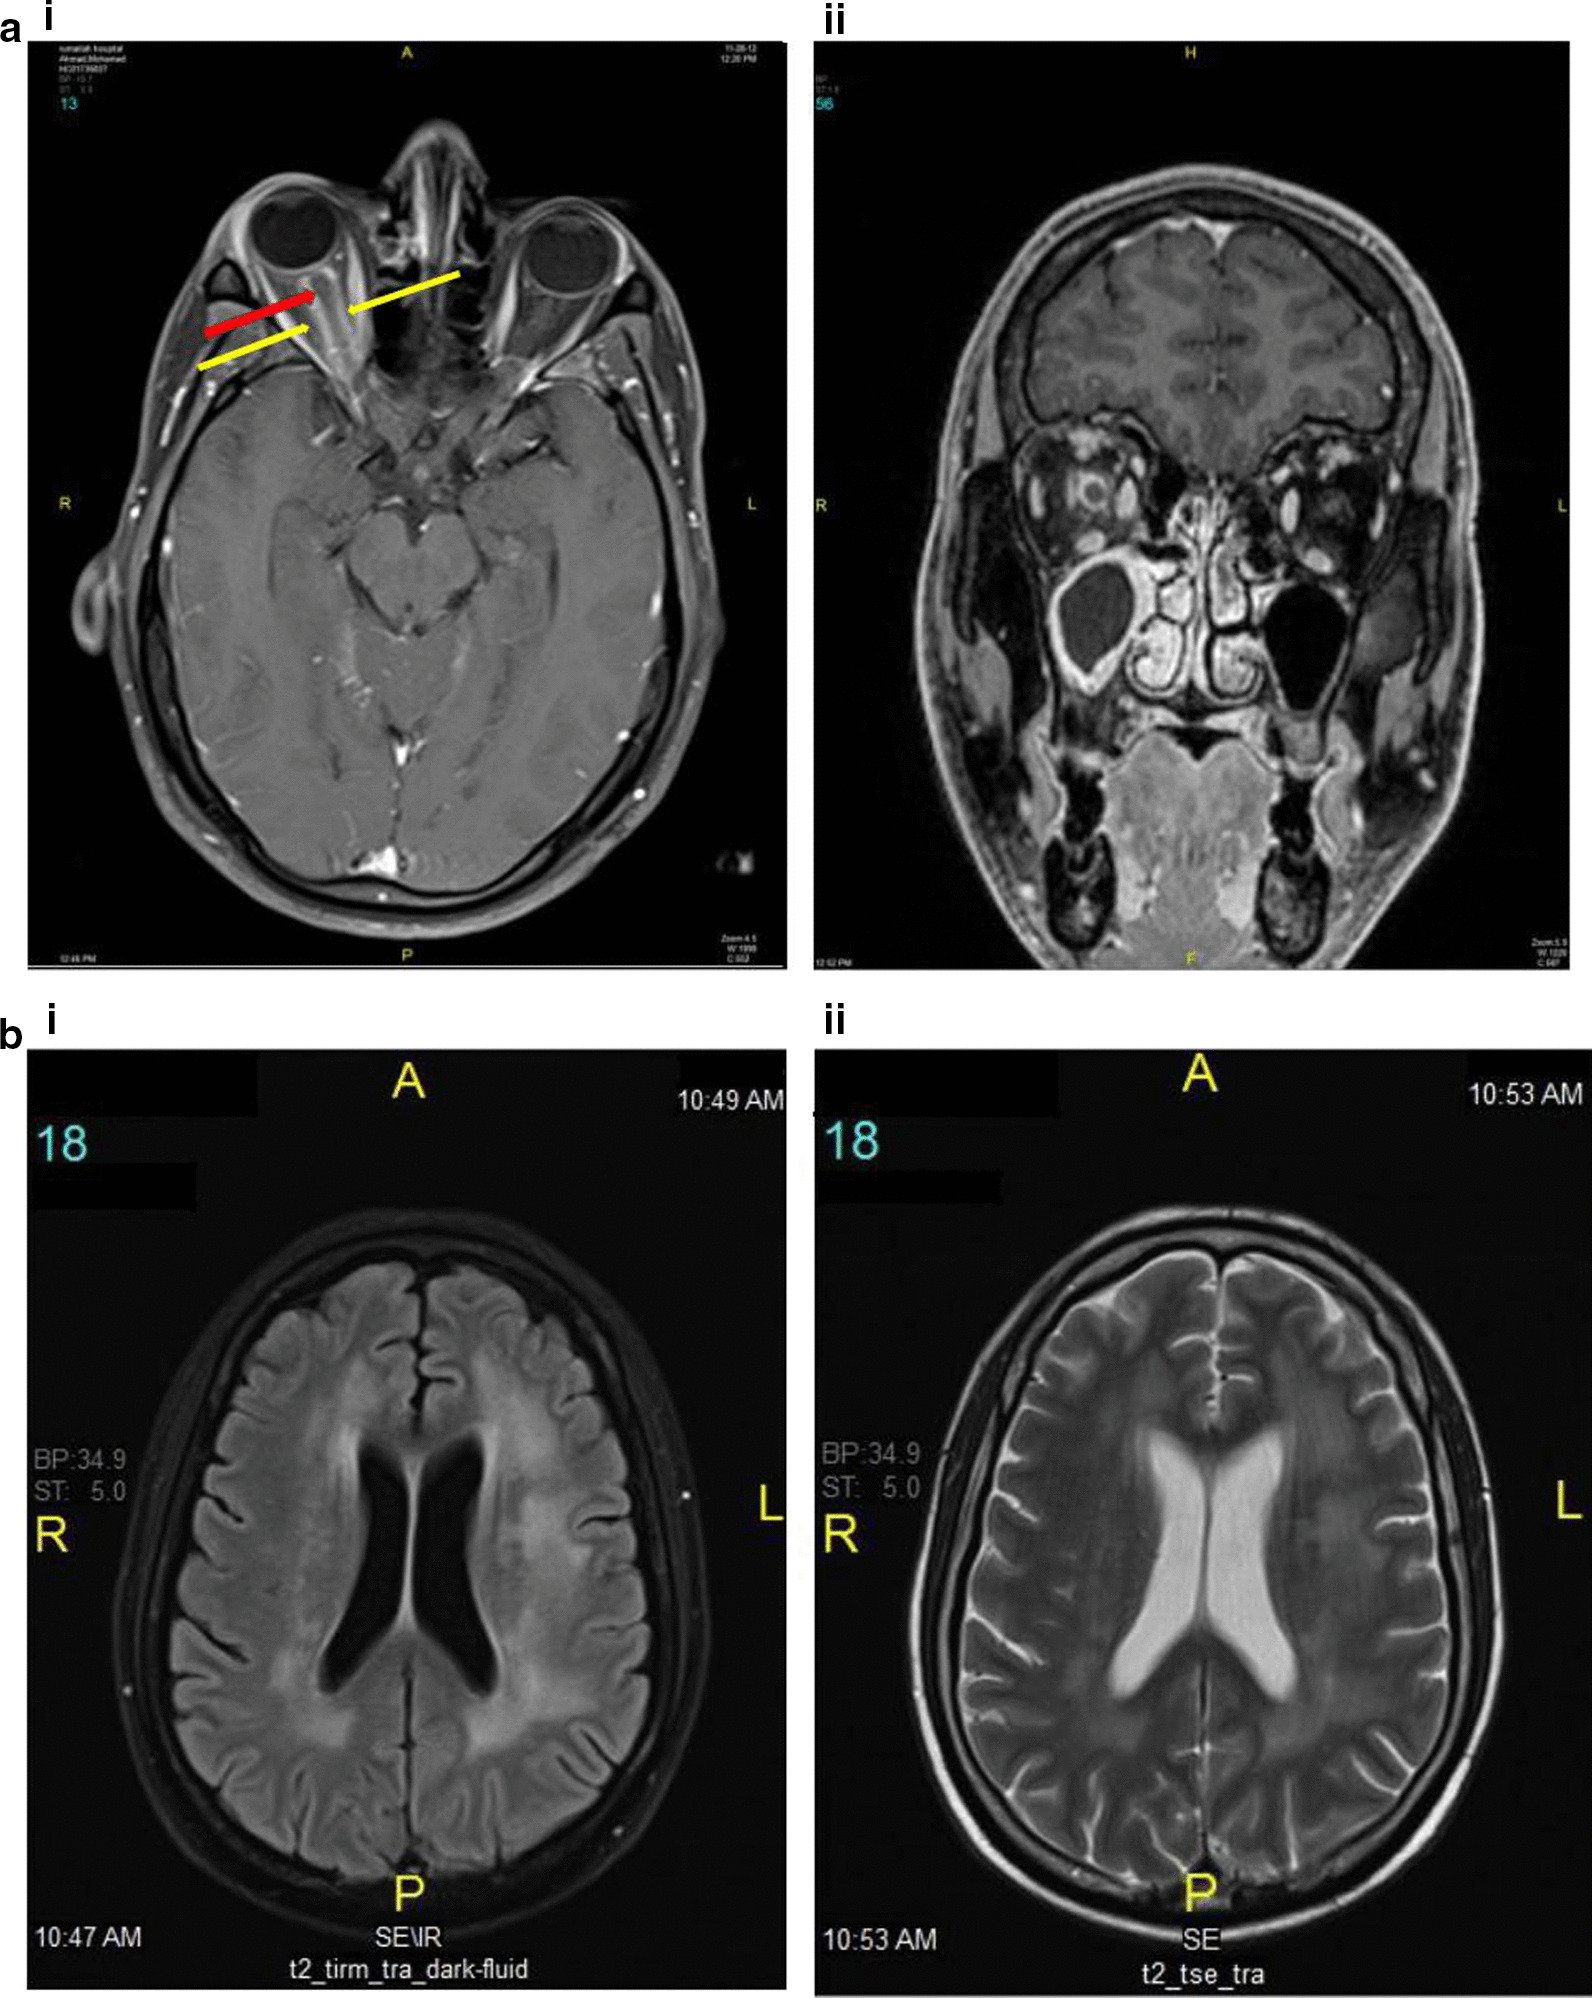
Case 2, radiological findings. a MRI brain at presentation. The right optic nerve is thicker than the left one (red arrow). Abnormal enhancement of the optic nerve sheath (tram-track sign) (yellow arrows) extends to the intraorbital and intracanalicular segments of the nerve. There is abnormal enhancement and stranding of the perioptic fat. Axial (i) and coronal (ii) T1-weighted post-contrast fat-saturated images are shown. b MRI brain at clinical progression. Newly developed multiple lesions in the periventricular white matter are noted. Two representative axial T1-weighted post-contrast fat-saturated images are shown
